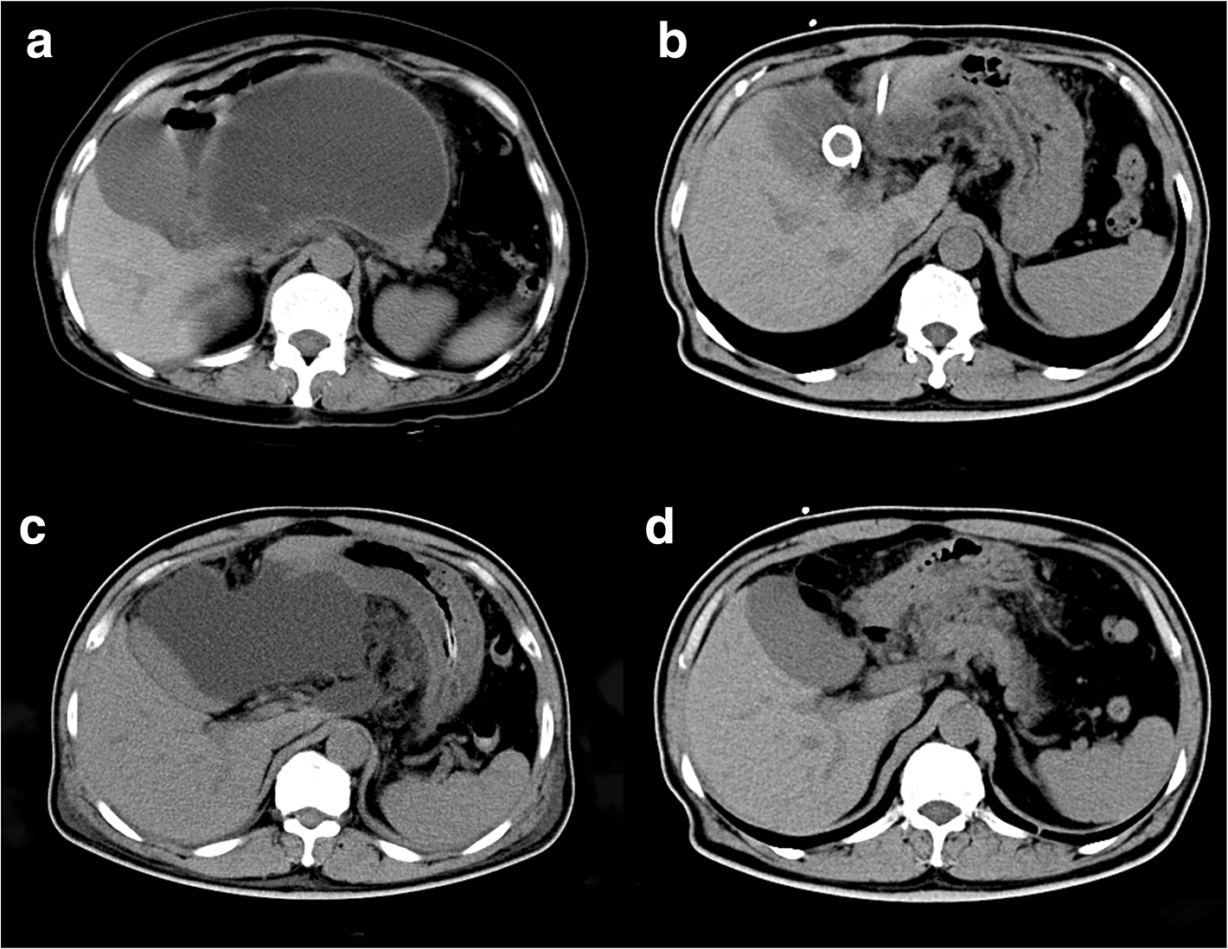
A PPC case who first received percutaneous drainage recurred, then was cured by surgical drainage. a Abdominal CT scan showing a 13-cm PPC; b PPC resolution on day 7 post-percuataneous drainage; c PPC recurrence in 2 months since percutaneous drainage; d PPC disappeared in 1 month since surgical drainage
